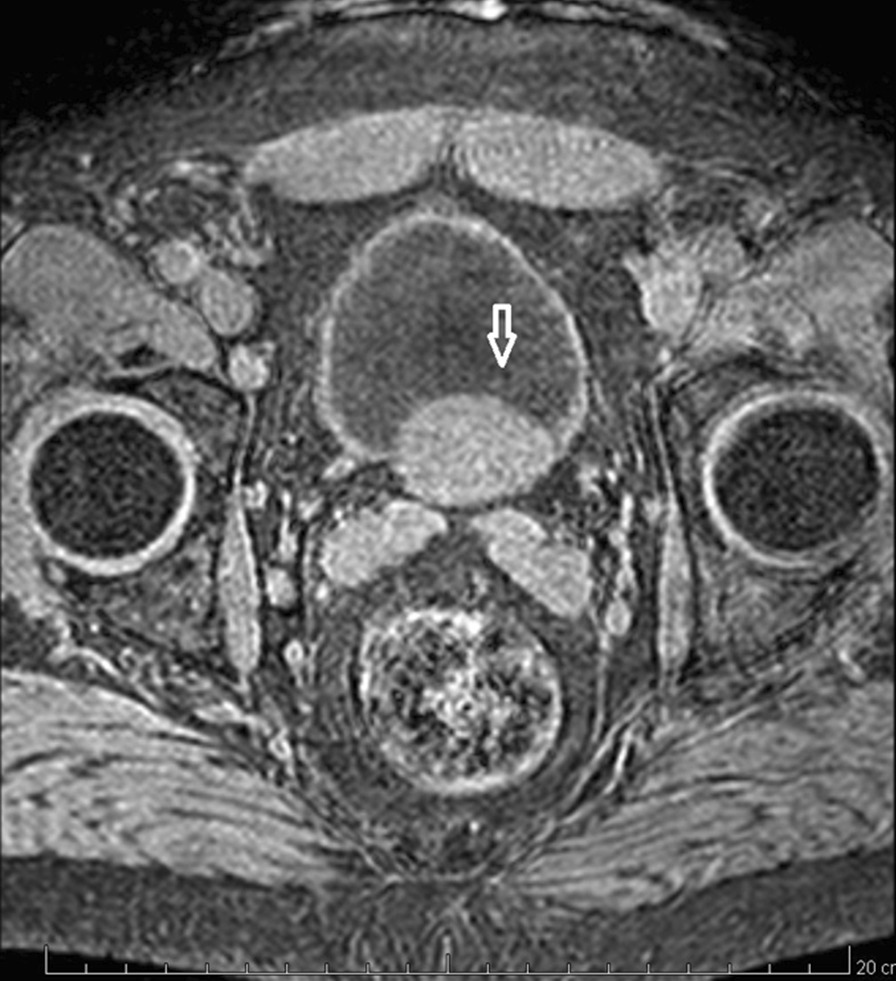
MRI revealed a well-defined 41 × 24 mm intramural mass
